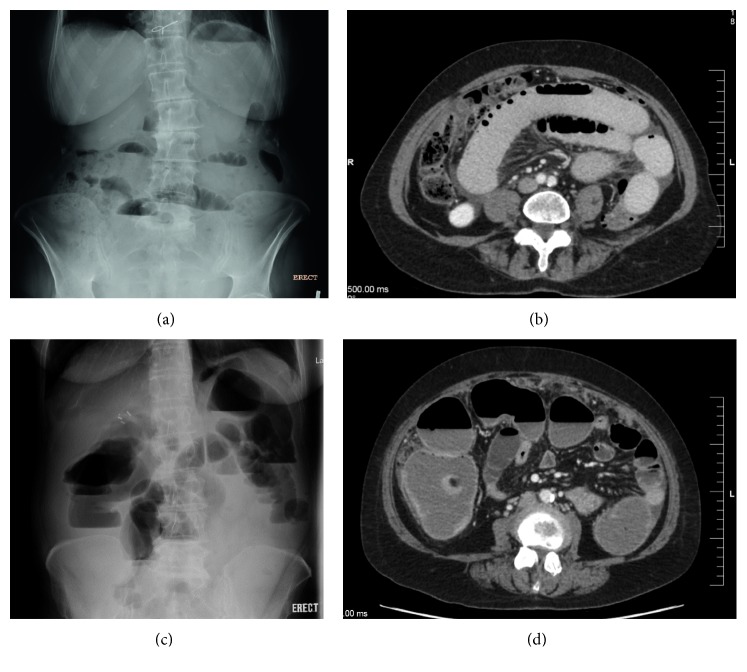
Radiographic images showing malignant bowel obstruction. (a) Abdominal radiograph in upright position showing multiple air-fluid levels consistent with small bowel obstruction (SBO). (b) Computed tomography (CT) confirms a high-grade SBO. (c) Abdominal radiographs in upright position showing large bowel obstruction (LBO). (d) CT demonstrates distended and fluid-filled large bowel loops concordant with LBO.
